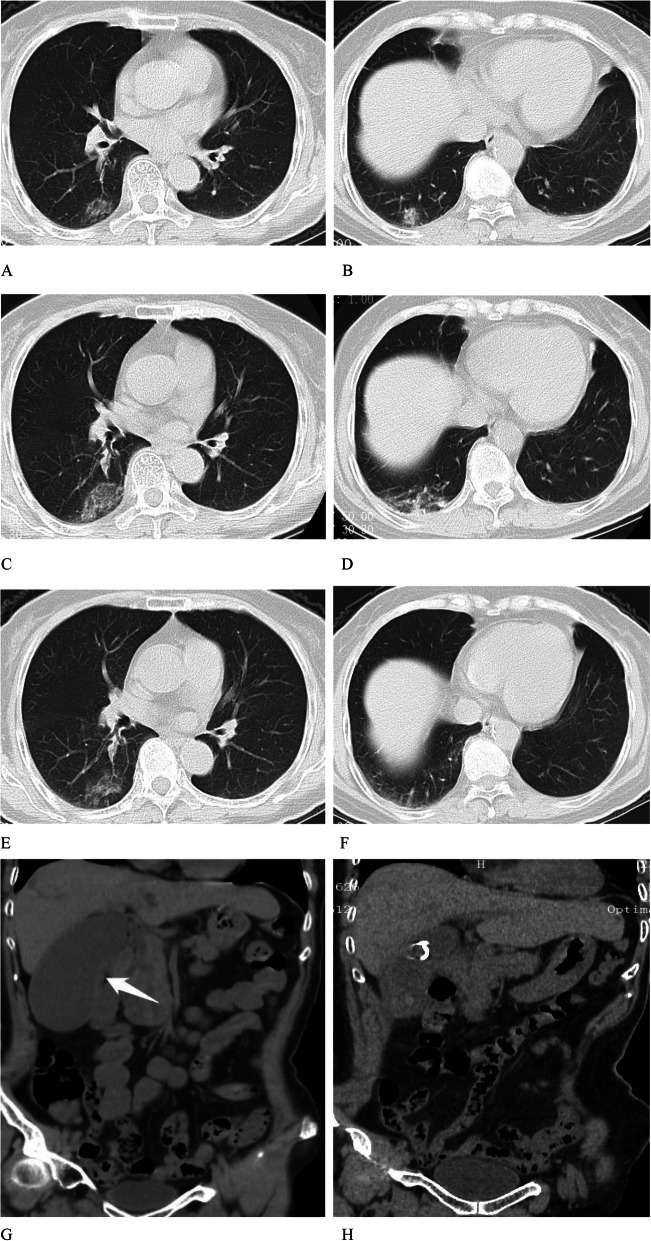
Chest and abdominal plain CT scans in a 68-year-old woman with COVID-19 pneumonia and acute csholecystitis. a, b. Transverse chest CT scan obtained on the first day after admission shows multifocal peripheral GGOs in the dorsal (a) and posterior basal (b) segments of the right lower lobe. c, d. Follow-up chest CT scan obtained on day 3 after admission shows progression of the GGOs. e, f. Follow-up chest CT scan obtained on day 13 after admission shows that the GGOs were partly resolved in the dorsal segment of the right lower lobe (e) and completely resolved in the posterior basal segment of the right lower lobe (f). g. Coronal MPR of the plain abdominal CT obtained on day 11 shows a distended gallbladder, hyperplasia of the gallbladder wall, biliary sludge (arrow), and no gallstones in the gallbladder. h. Coronal MPR of the plain abdominal CT obtained on day 13 shows shrinkage of the gallbladder or its change in shape, a high-density drainage tube and a little bleeding in the gallbladder. CT = computer tomography, GGOs = ground-glass opacities, MPR = multiplanar reconstruction
